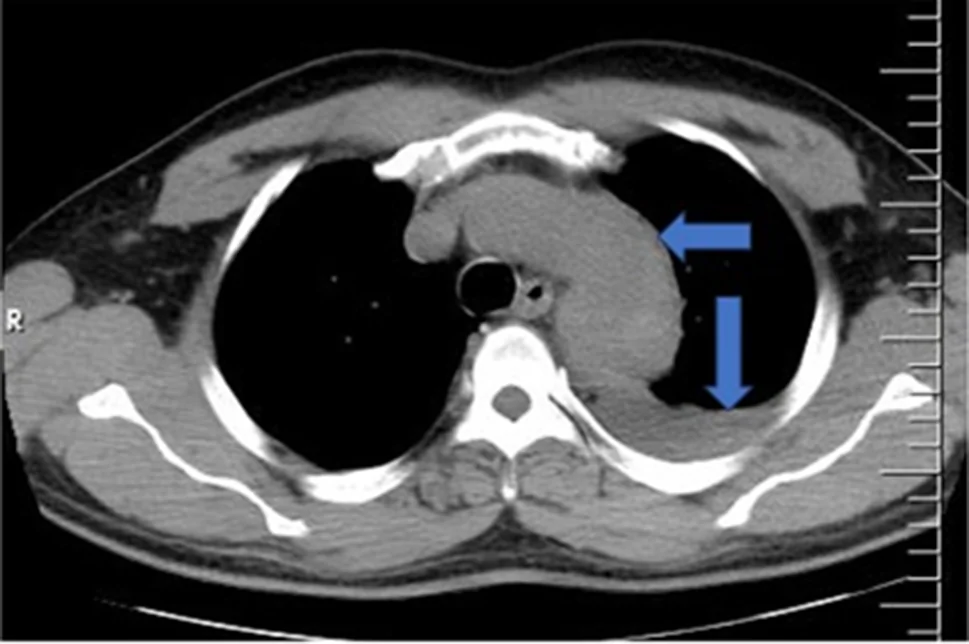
Pre-contrast axial chest CT image at the level of the aortic arch demonstrating significant aneurysmal dilation (horizontal arrow) and a left-sided pleural effusion (arrow pointing downward), often a sign of periaortic inflammation or incipient rupture in the setting of AAD
